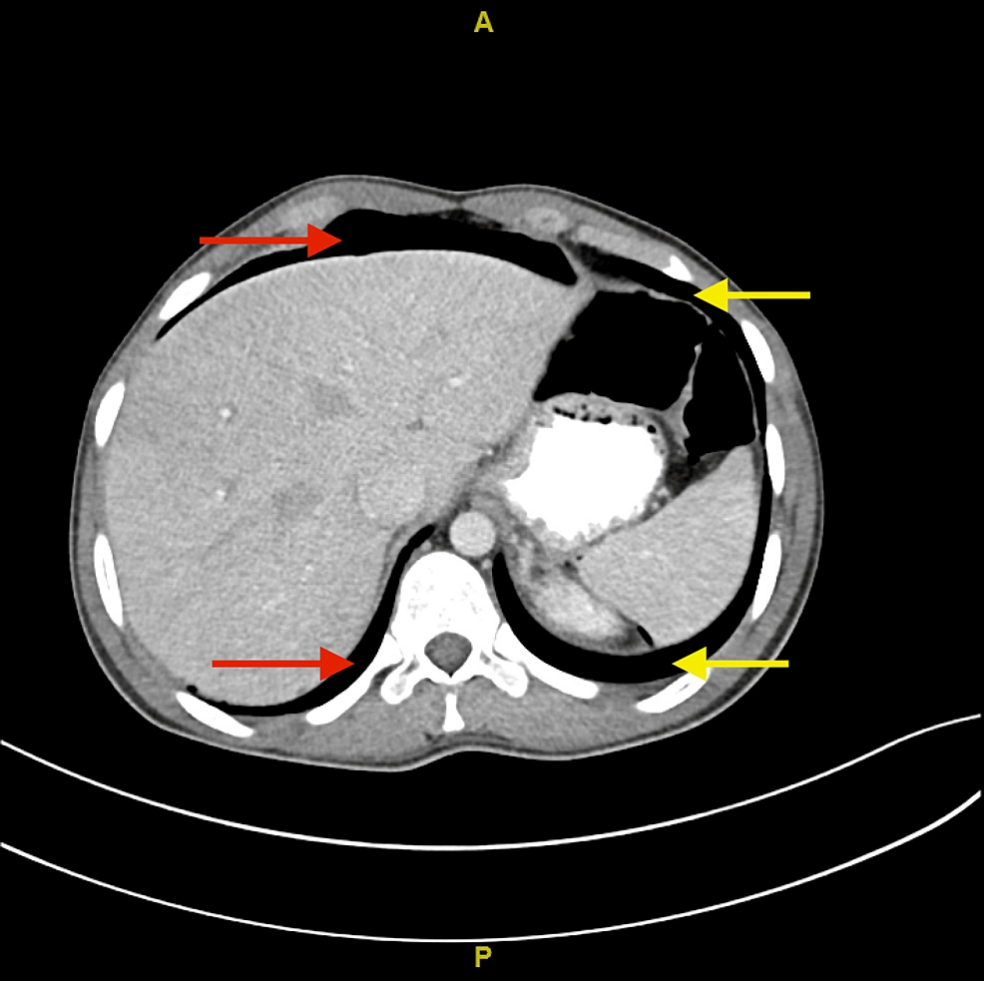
Axial CT of the abdomen. The red arrow shows air in the peri-hepatic area. The yellow arrow shows air in the peri-splenic area.
